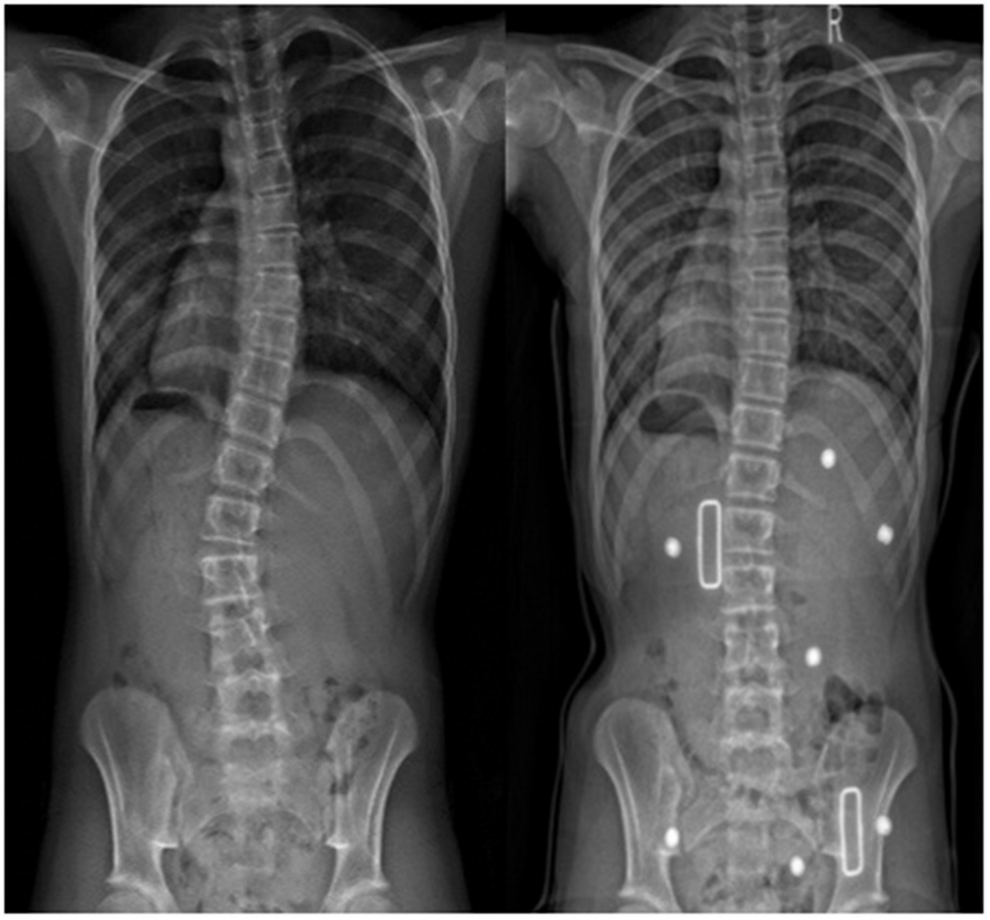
Pre-brace (left) and in-brace radiographs (right) of a participant.
